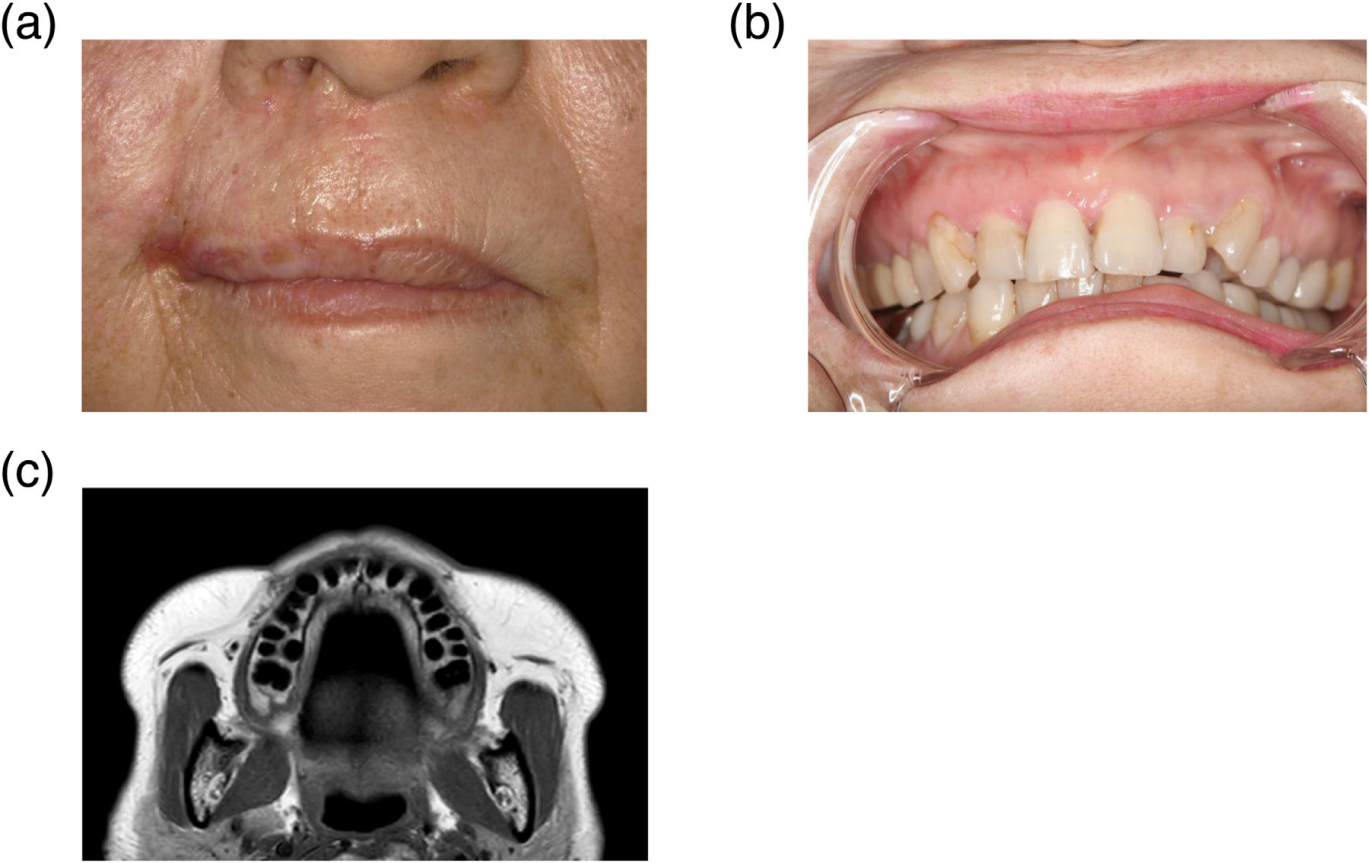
Clinical results 8 years after carbon-ion radiation therapy. (A) Skin findings. (B) Oral findings. (C) Maxillary bone findings on T1-weighed axial magnetic resonance images.
